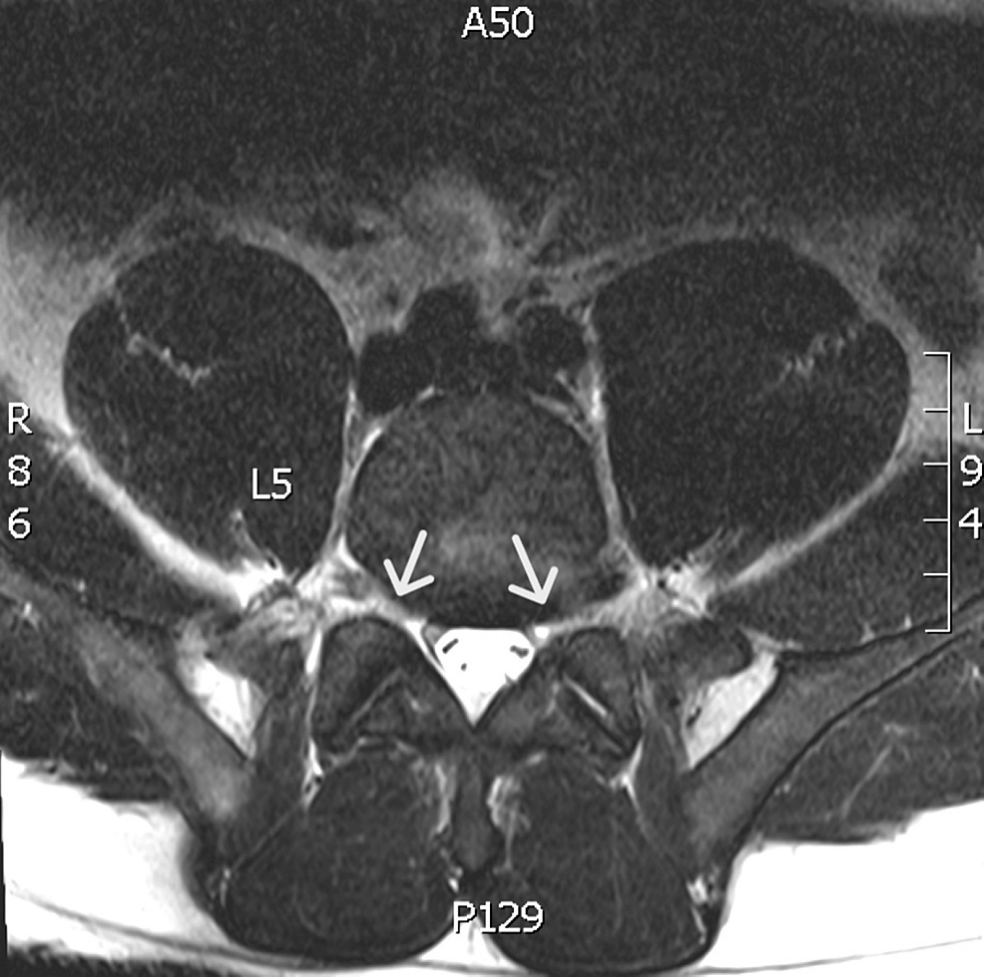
T2 weighted MRI demonstrating mild bilateral neural foraminal narrowing at L5-S1, shown with arrows.
